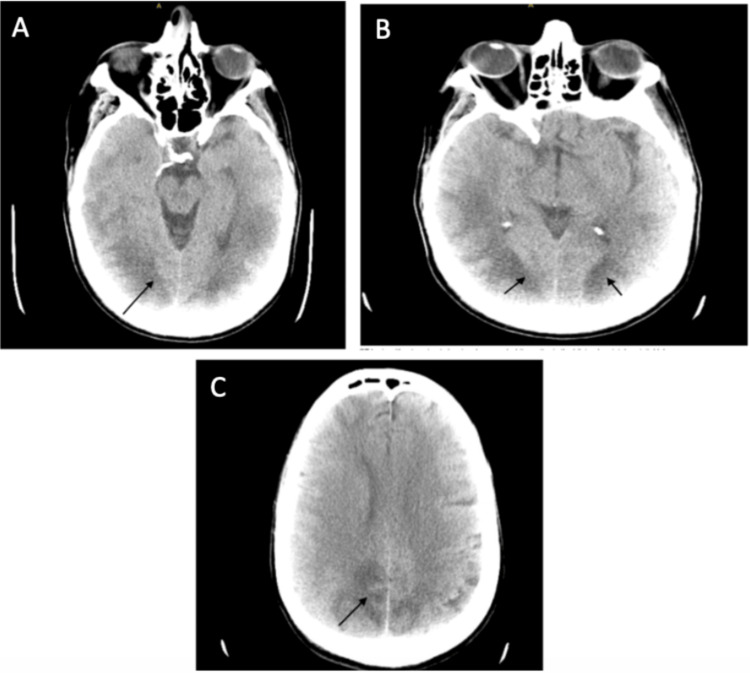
Non-contrast computed tomography of the brain. Axial non-contrast computed tomography images demonstrate decreased white matter attenuation in the parieto-occipital lobes bilaterally (arrows). (A) Axial slice at the basal ganglia level showing early posterior hypodensity. (B) Axial slice at the ventricular level demonstrating bilateral parieto-occipital white matter changes. (C) Superior axial slice confirming persistent posterior white matter hypodensity.
